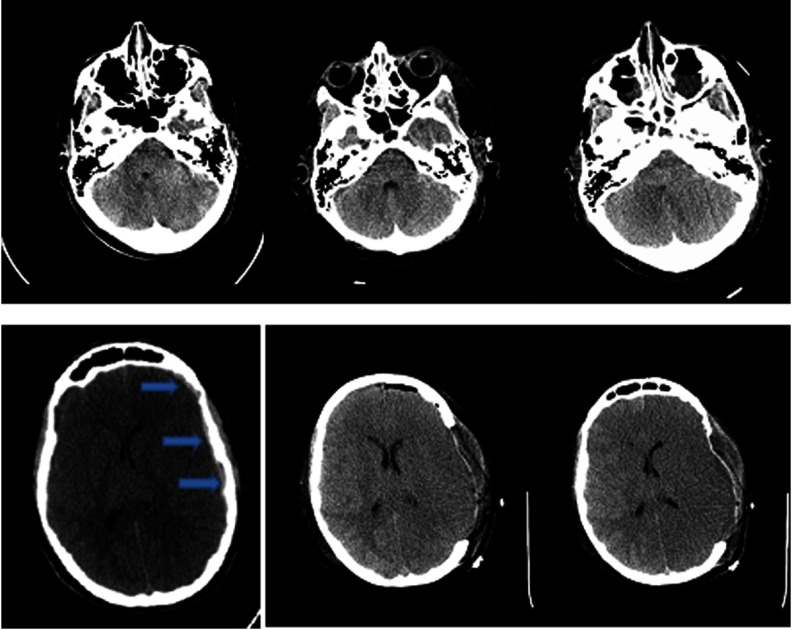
Non-contrast brain CT 2 hours after the patient’s injury (column A), 2 days post-injury (column B), and 3 days post-injury (column C). (A) There is generalized cerebral edema, moreso on the left side, with effacement of the sulci, uncal herniation, 3 mm midline shift to the right, and a small left-sided SDH approximately 2–3 mm thick (arrows). (B) Two days after left decompressive craniectomy with persistent generalized cerebral edema and interval resolution of the midline shift and signs of uncal herniation, and drainage of the SDH. (C) Progression of cerebral edema with complete absence of gray–white matter differentiation.
